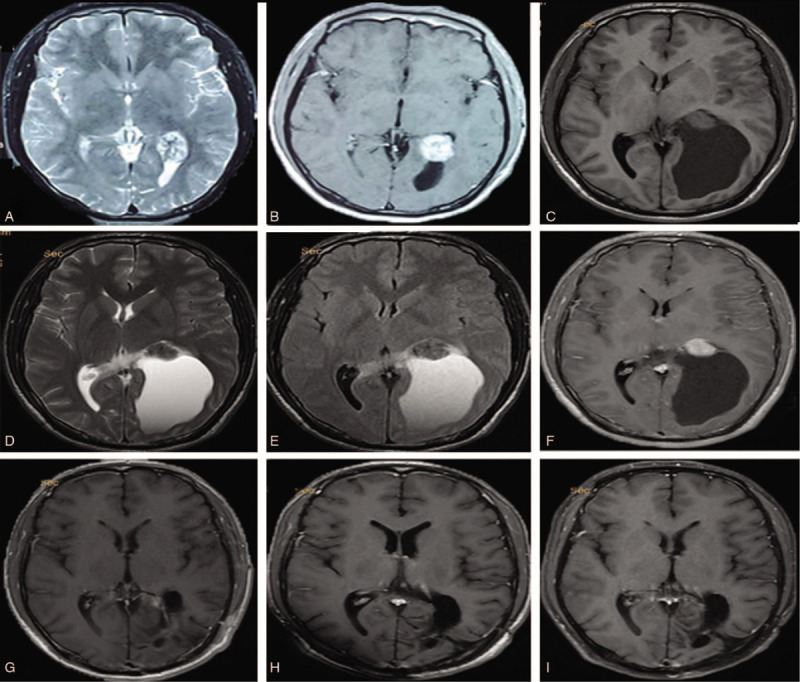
Magnetic resonance imaging revealing a cystic-solid mass in the left lateral ventricle trigone. (A) Magnetic resonance imaging (MRI) T2 sequence was performed on July 10, 2013. (B) Enhanced MRI was performed on August 12, 2014, which revealed that the tumor parenchyma was homogeneously enhanced. (C–F) MRI was performed 1 wk before operation on July 29, 2017, which suggested cystic changes in the tumor (C: T1; D: T2; E: T2-FLAIR; F: enhanced). (G–I) Postoperative enhanced MRI showed no tumor growth (G: 6 mo after surgery; H: 1 yr after surgery; I: 2 yr after surgery).
